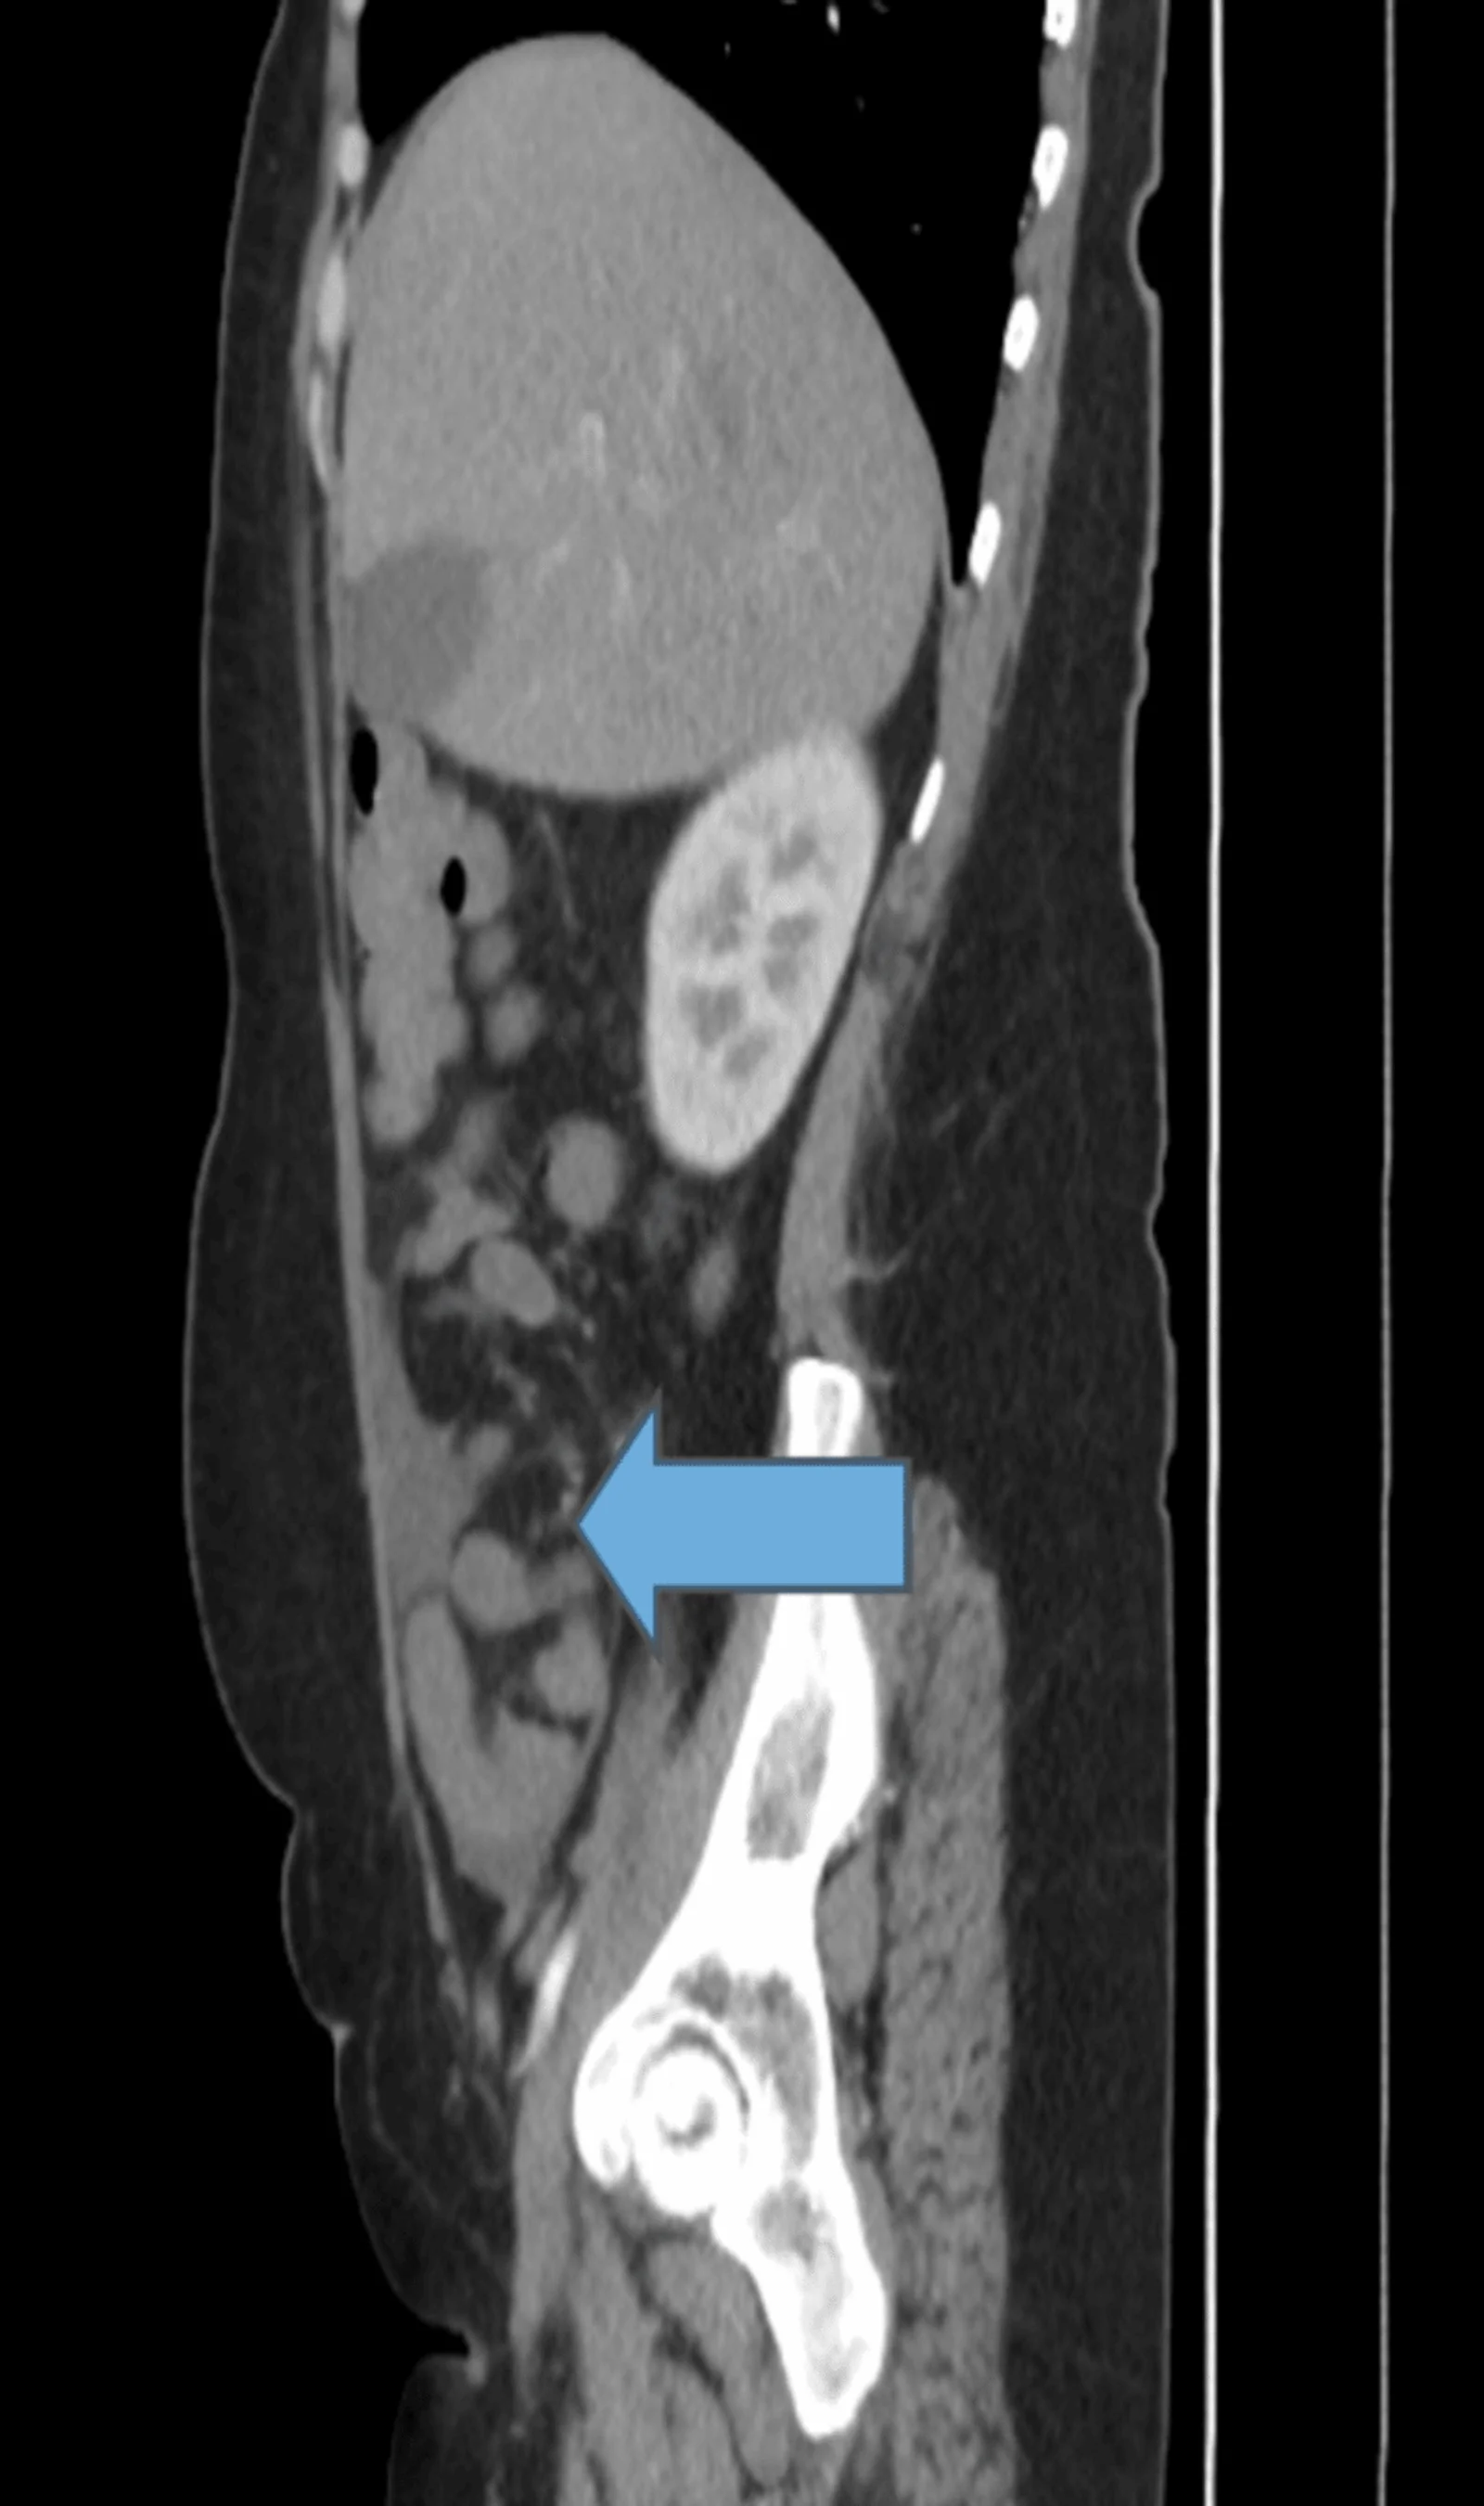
Sagittal Contrast-Enhanced Abdominal CT Image Sagittal contrast-enhanced CT view demonstrating the posterior demarcation of the cocoon sac (arrow). A small amount of intraperitoneal fluid was also identified. CT: computed tomography
